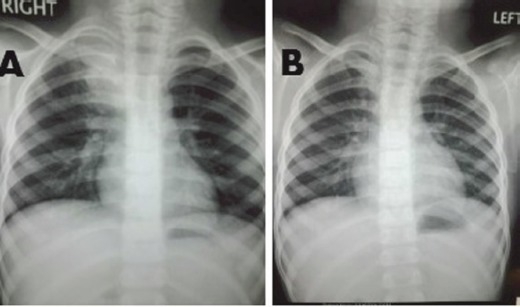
Panel A: Chest x-ray showing inhomogeneous opacity in the right upper lobe of lung. Panel B: Resolution of the inhomogeneous opacity following chemotherapy and radiation.
